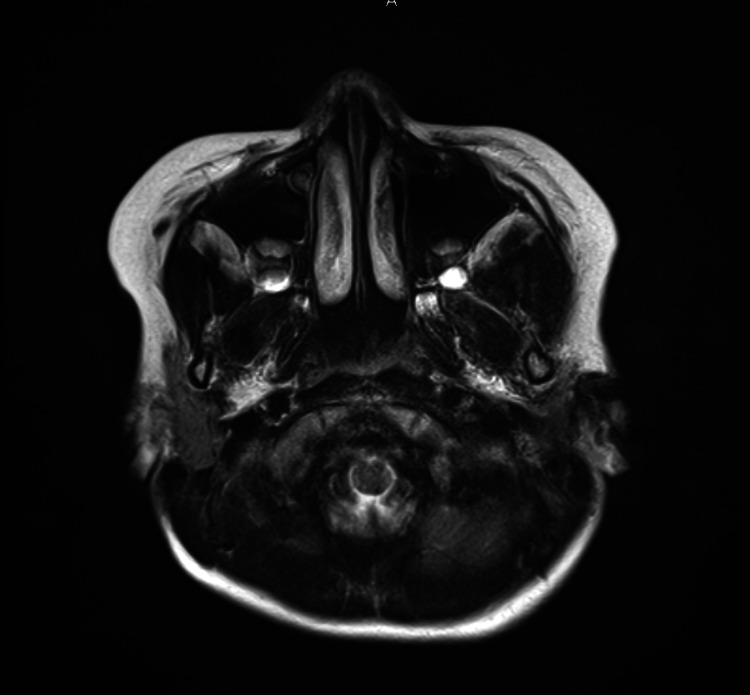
Imaging of Case 1: temporal MRI (T2-weighted image) MRI, magnetic resonance imaging.
